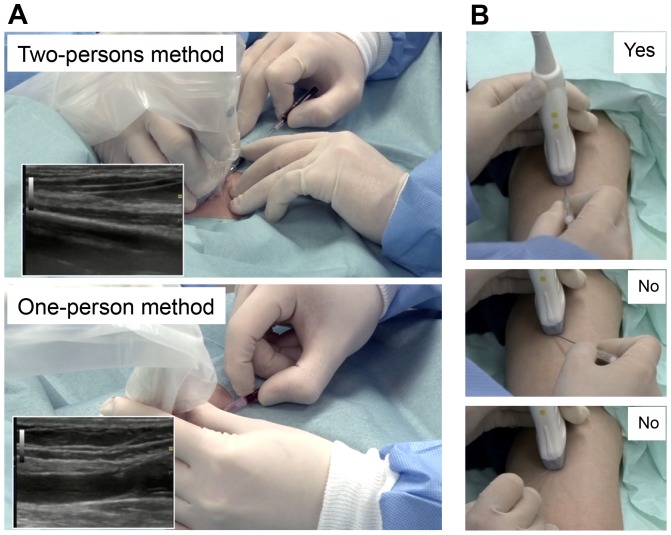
Real-time ultrasound-guided venipuncture. (A) “Two-person method” and “one-person method.” With the “two-person method,” the ultrasound-guidance step and the puncturing step are assigned separately to two operators. This can increase the success rate for beginners. (B) Tips for puncture with the correct angle. The axes between the probe and needle must be kept in one line.
